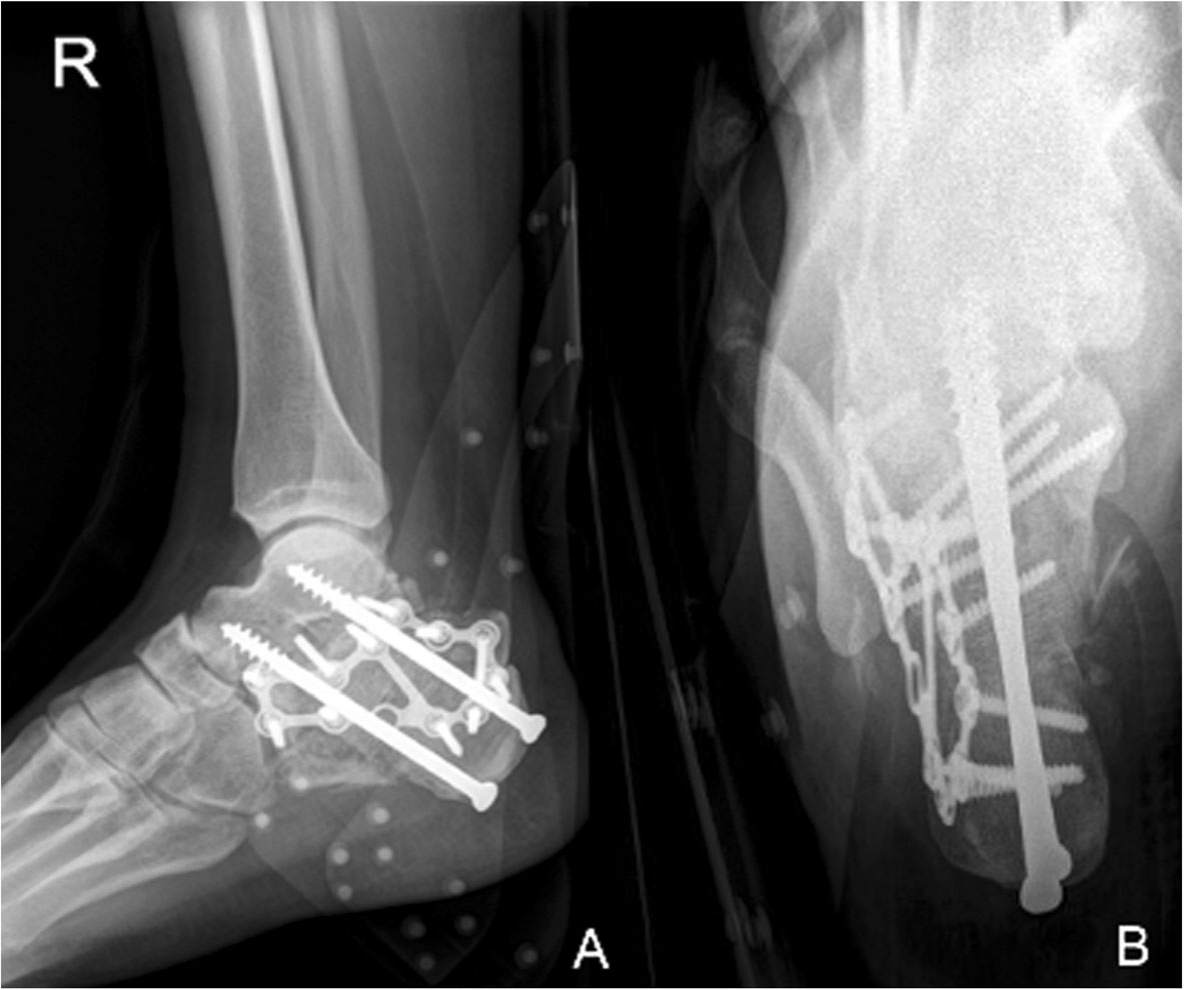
Case 2 Postoperative radiographs Lateral (A) and axial (B) radiographs after primary subtalar arthrodesis
